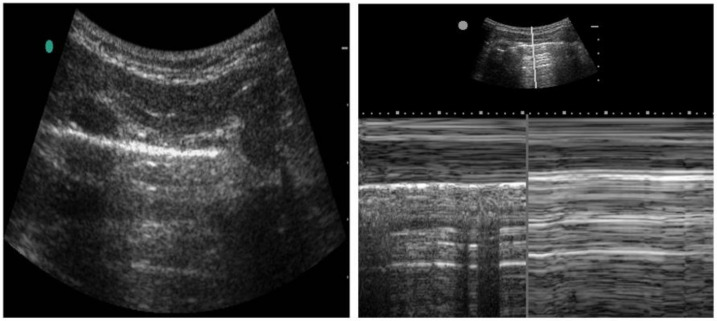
Ultrasound assessment for the pneumothorax. B-mode (Left) and M-mode (Right) technique for assessing pneumothorax. The B-mode demonstrates a normal visceral pleura lining (horizontal white line). The M-mode image demonstrates a normal pleura motion (Left) and abnormal pleural motion or ‘stratosphere sign’ (Right). The abnormal pleural motion is indicative of pneumothorax.
